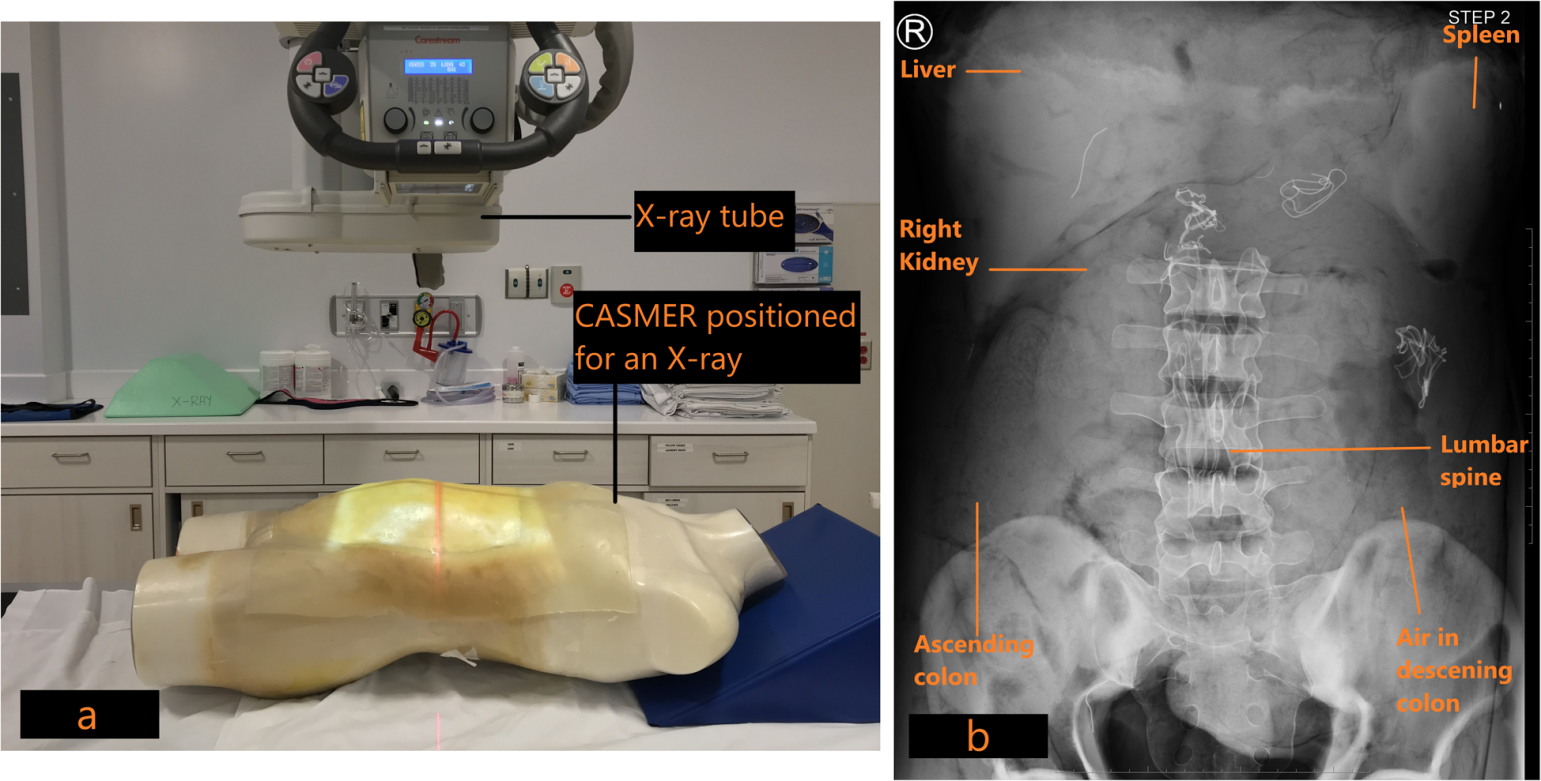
a: CASMER was positioned for an anteroposterior abdominal radiograph to determine radiological density. b: Anteroposterior X-ray of CASMER demonstrates the 3D printed organs and other structures as labelled
